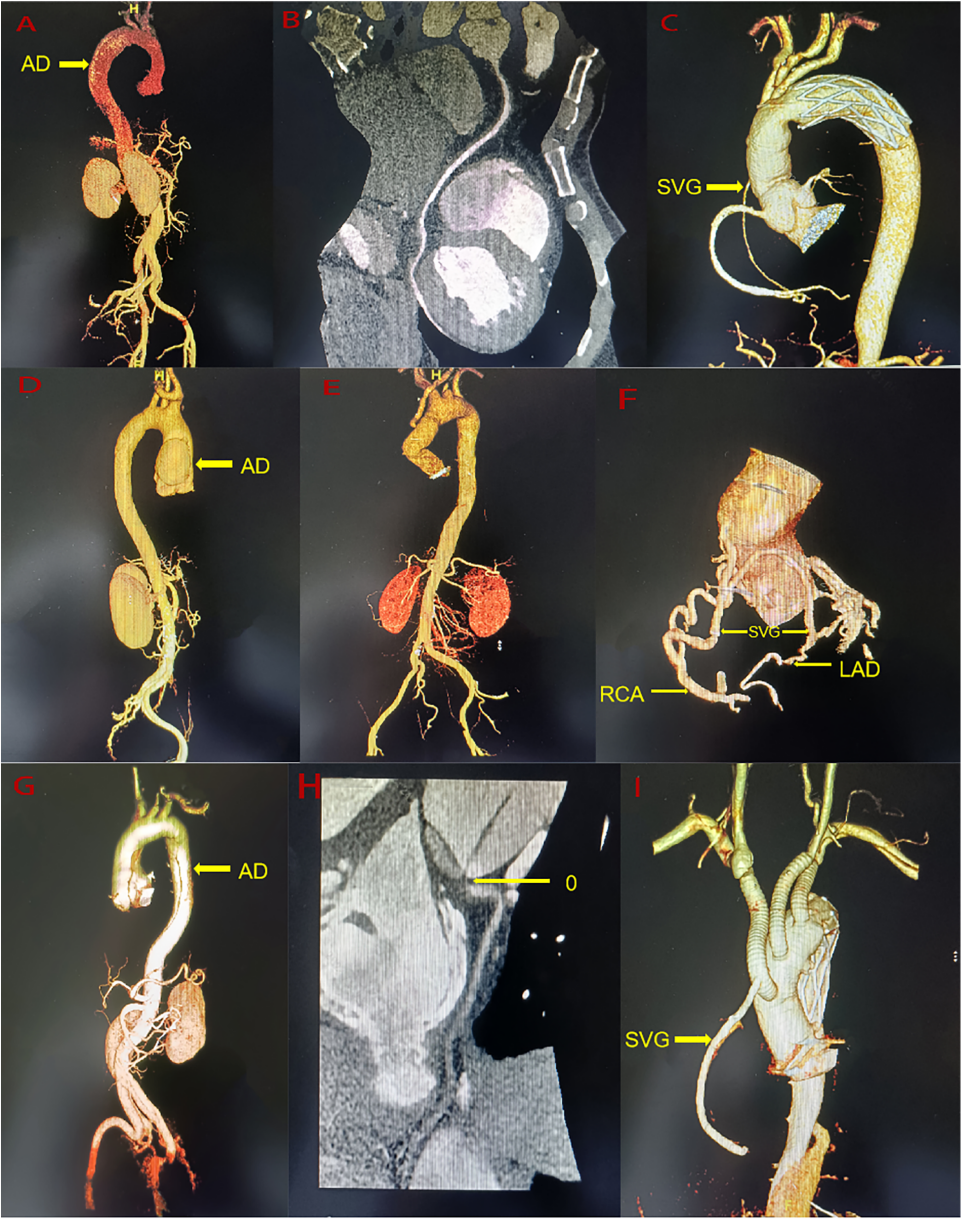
(A) A pre-operative the entire aorta CTA of case 1. (B) A pre-operative the right coronary artery CTA of Case 1. (C) A post-operative the entire aorta CTA of Case 1. (D) A pre-operative the entire aorta CTA of Case 2. (E) A post-operative the entire aorta CTA of Case 2. (F) A post-operative the coronary artery CTA of Case 2. (G) A pre-operative the entire aorta CTA of Case 3. (H) A pre-operative the right coronary artery CTA of Case 3. (I) A post-operative the entire aorta CTA of Case 3. AD, aortic dissection; RAC, right coronary artery; LAD, anterior descending branch; SVG, saphenous vein graft.
